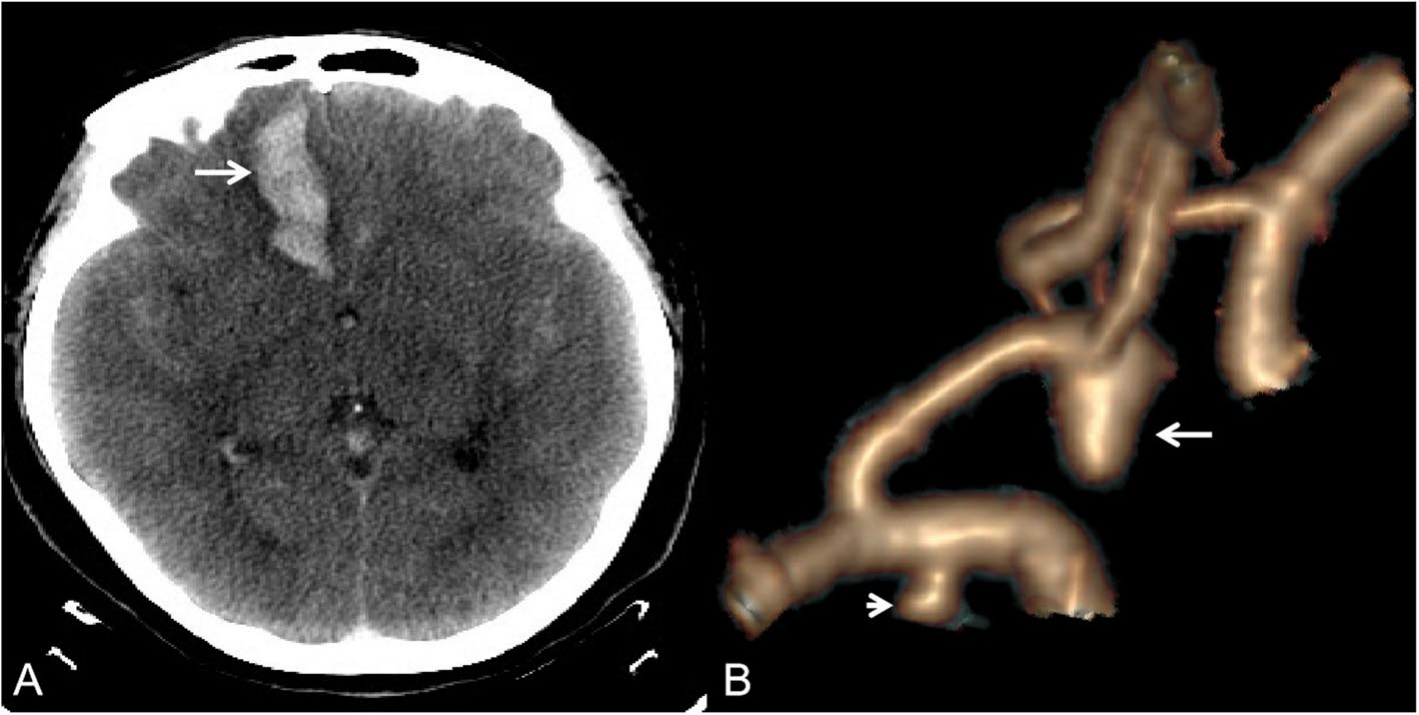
A 52-year-old female presented with severe headache. CT scan showed subarachnoid hemorrhage with a focal hematoma (arrow). Computed tomography angiography showed a ruptured anterior communicating artery aneurysm (large arrow, aneurysm-specific prediction score = 0.90592) and a unruptured internal carotid artery aneurysm (small arrow, aneurysm-specific prediction score = -0.42223)
